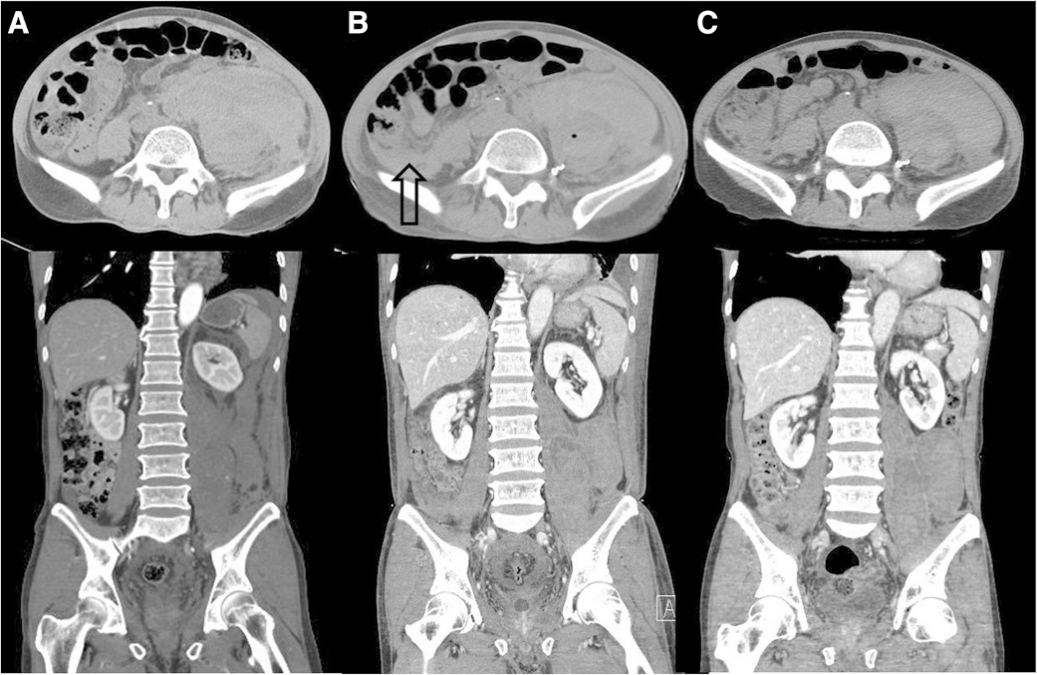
Serial abdominal CT. a: Initial abdominal CT; b: Five days after TAE, the arrow indicates the newly developed right iliopsoas hematoma; c: Final follow-up CT, CT : computed tomography, TAE : transarterial embolization
